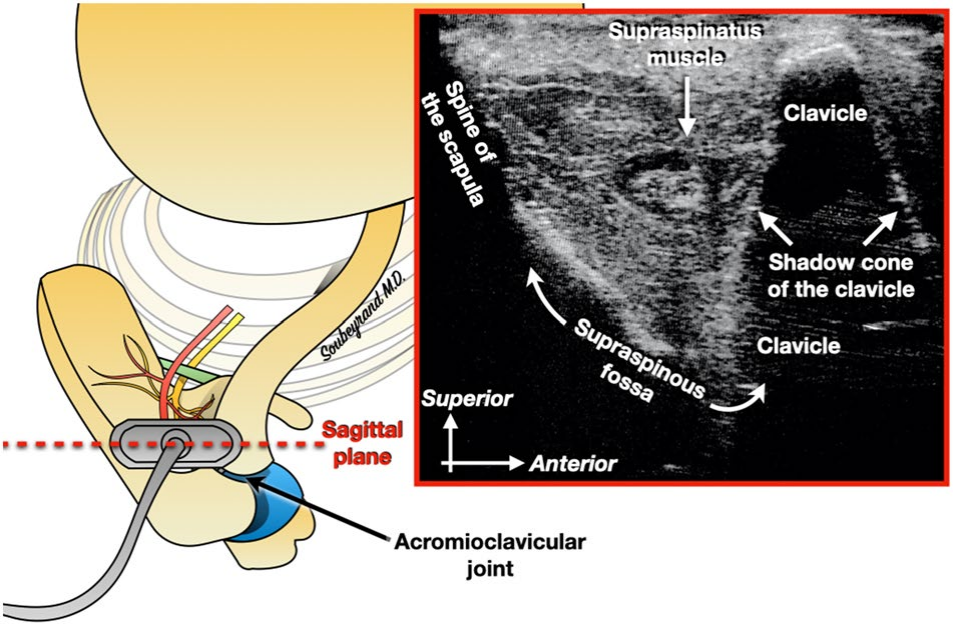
The ultrasound probe is positioned in the sagittal plane, just behind the acromioclavicular joint. The supraspinous fossa can thus be identified.
